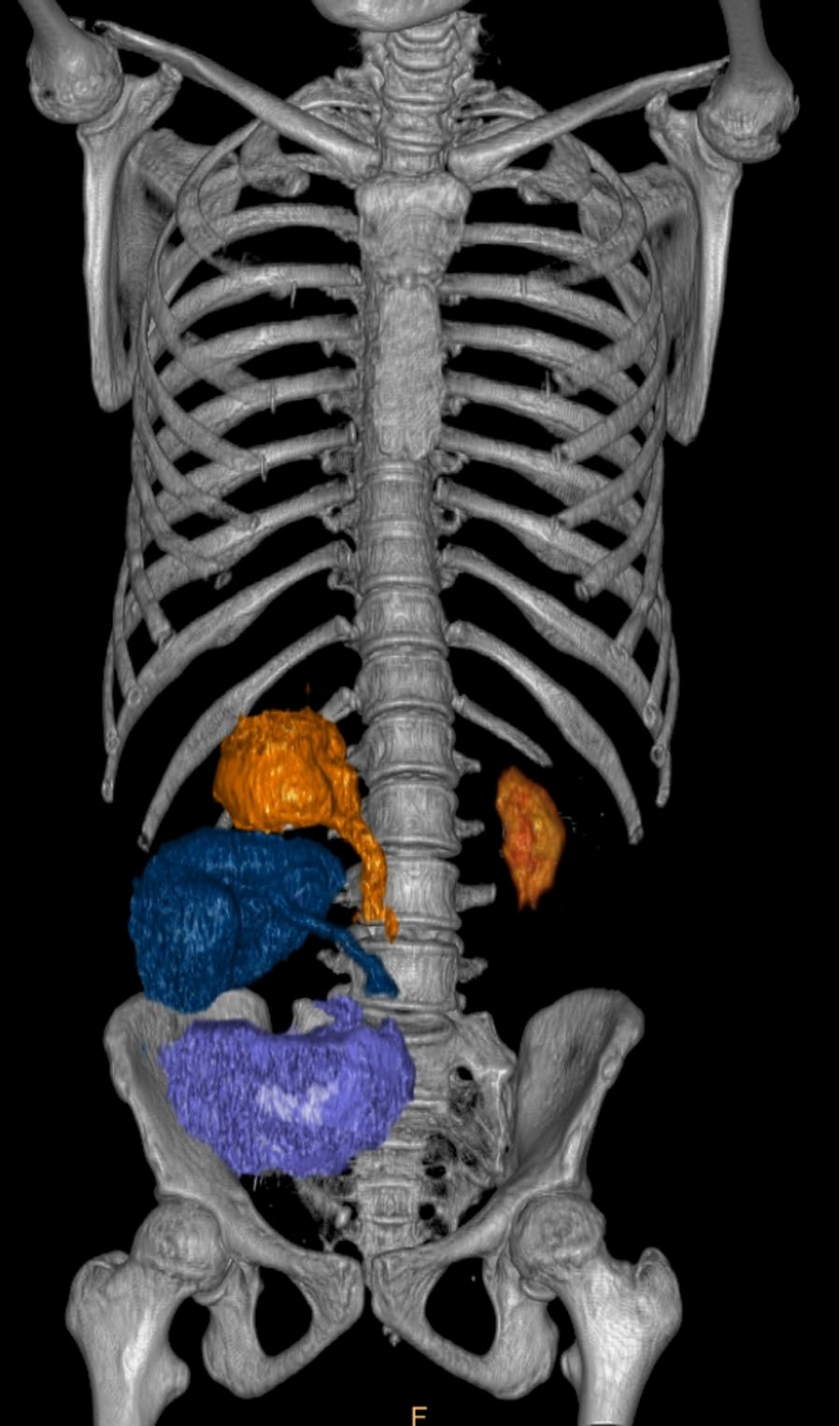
CT reconstruction showing the patient’s native kidneys (orange), the right flank transplanted kidney (blue) and the right iliac fossa transplanted kidney (violet)
